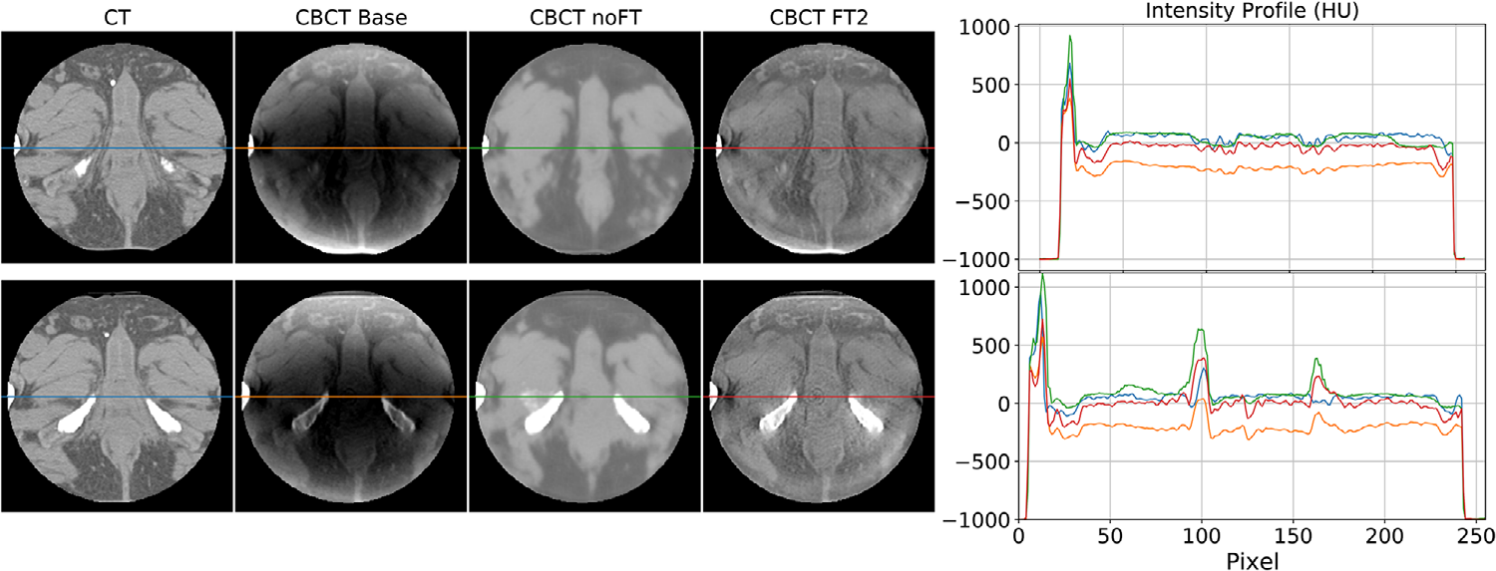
Comparison between a single CBCT Base and corresponding CT axial slice with the CBCT elaborated by noFT and FT${}_{2}$ models. The rightmost part of the figure compares the intensity profiles of the central line of the images, highlighted by the central line in the four representations. Images are displayed with Window = 400, Level = 20
